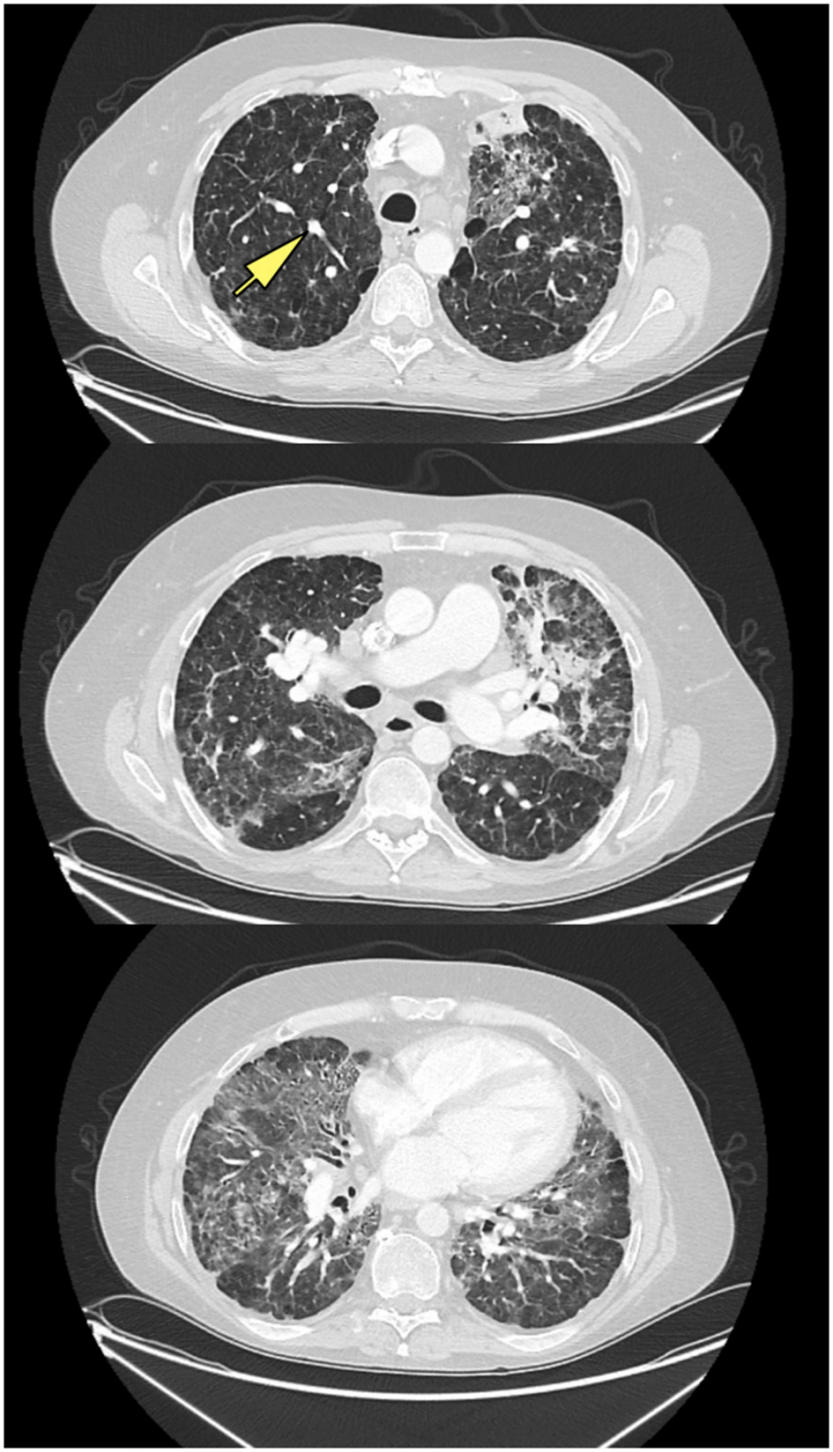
CT scan of ILD/CVID patient, with upper, middle, and lower lung zones, demonstrating mid to lower lung zone predominant ground glass opacities, within a bronchovascular distribution, with associated volume loss, with left upper lobe consolidation in association with air bronchograms, likely pneumonia, new solid nodular opacity (12 × 7 mm) (yellow arrow) in the right upper lobe, as well as bilateral hilar lymphadenopathy.
